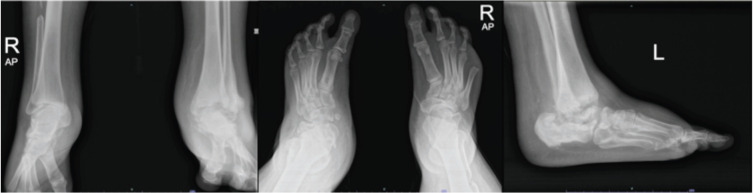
Radiographs image after four months treatment with pamidronate infusion.
